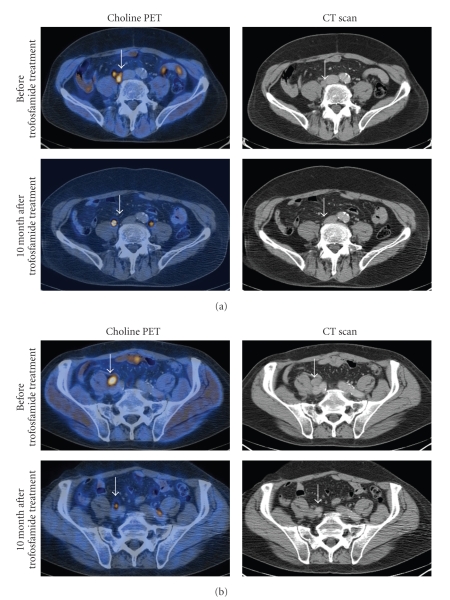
(a) and (b) show exemplarily the regression of a lymph node metastasis, demonstrated by choline-PET-CT before and 10 months after treatment with trofosfamide.
